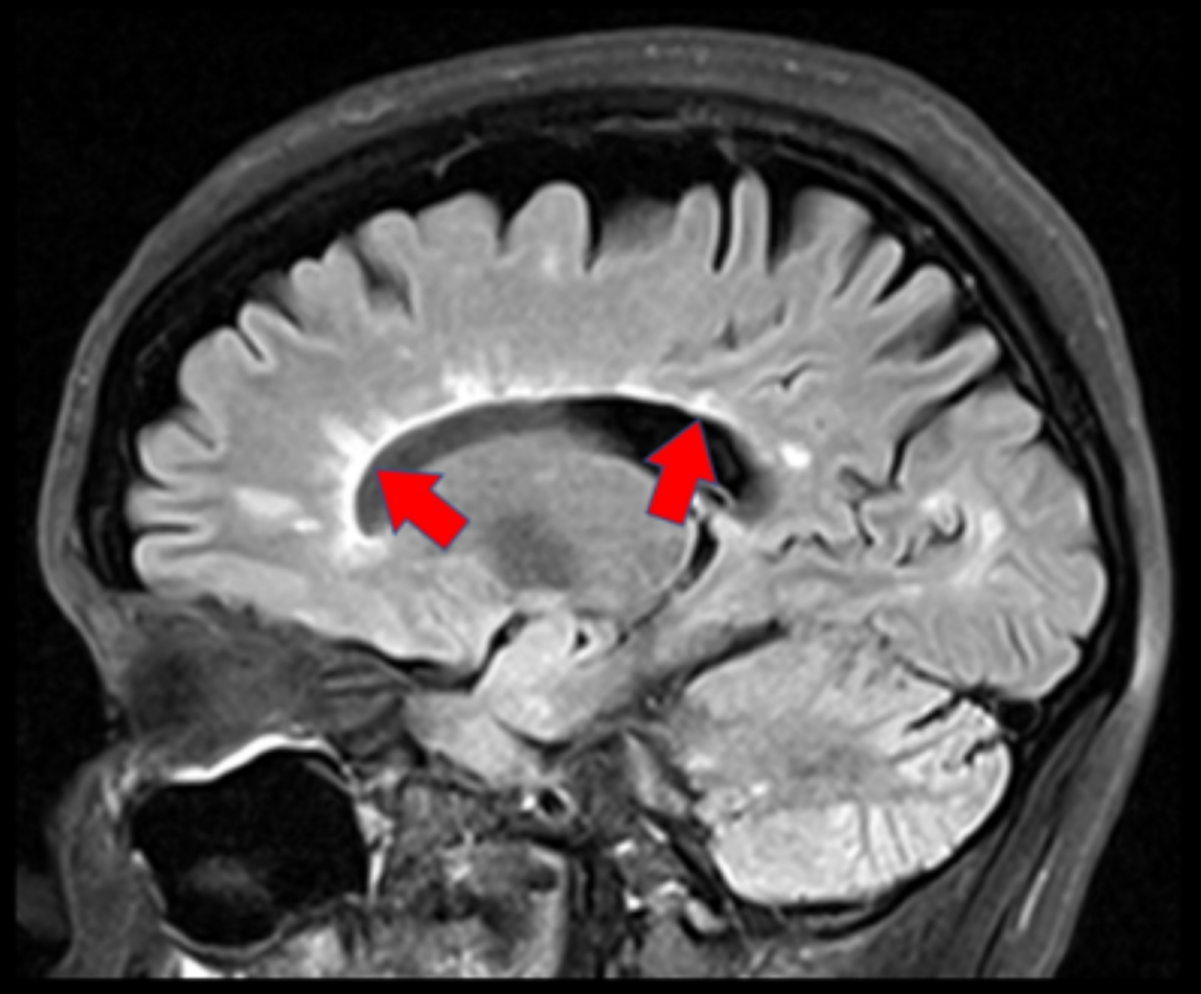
Sagittal view of the patient’s brain on MRI through the corpus callosum demonstrating multiple pericallosal finger-like projections of T2 hyperintensity representing demyelinating plaques known as Dawson fingers (red arrows).
